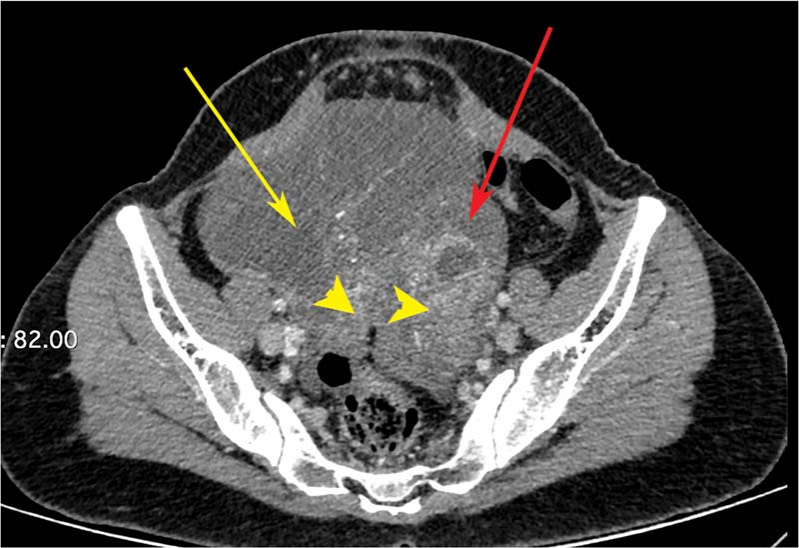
CT scan of the abdomen showing right ovarian cyst (yellow arrow), left ovarian cyst (red arrow) with solid elements within (arrow heads).
